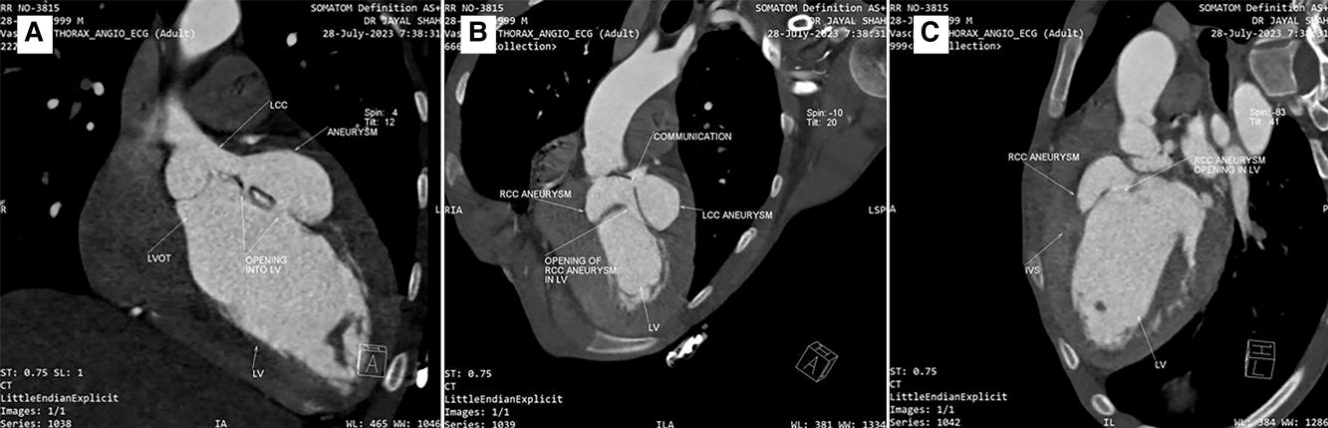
(*A*) Computed tomography showing aneurysm originating from the left coronary sinus and rupturing into the left ventricle through two separate openings. (*B*) Image showing the two aneurysms from the right and left coronary sinuses communicating with each other and the right sinus of Valsalva aneurysms rupturing into the left ventricle. (*C*) Image showing the sinus of Valsalva aneurysms from the right coronary sinus rupturing into the interventricular septum and opening into the left ventricle.
